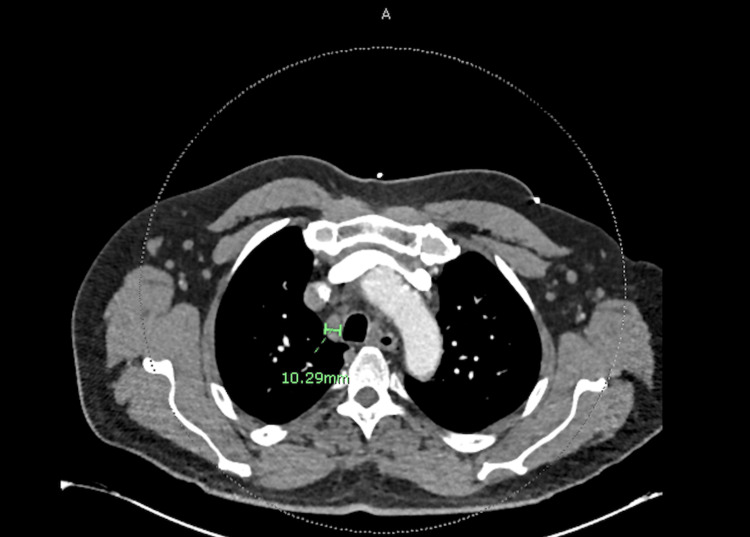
Cross section of contrast-enhanced CT scan showing 10.29 mm mediastinal lymph node
